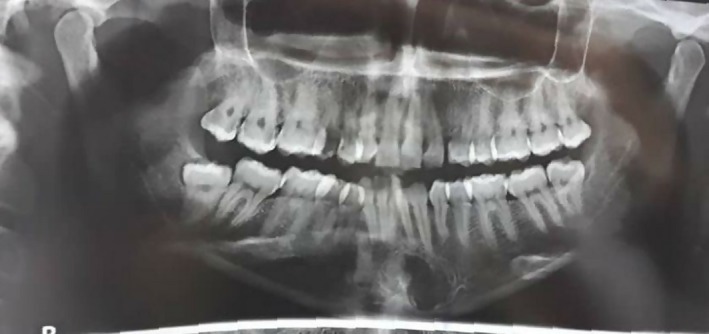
Radiograph of a patient with intraosseous lipoma. This is panoramic view which shows a unilocular lesion in the symphysis of the mandible
